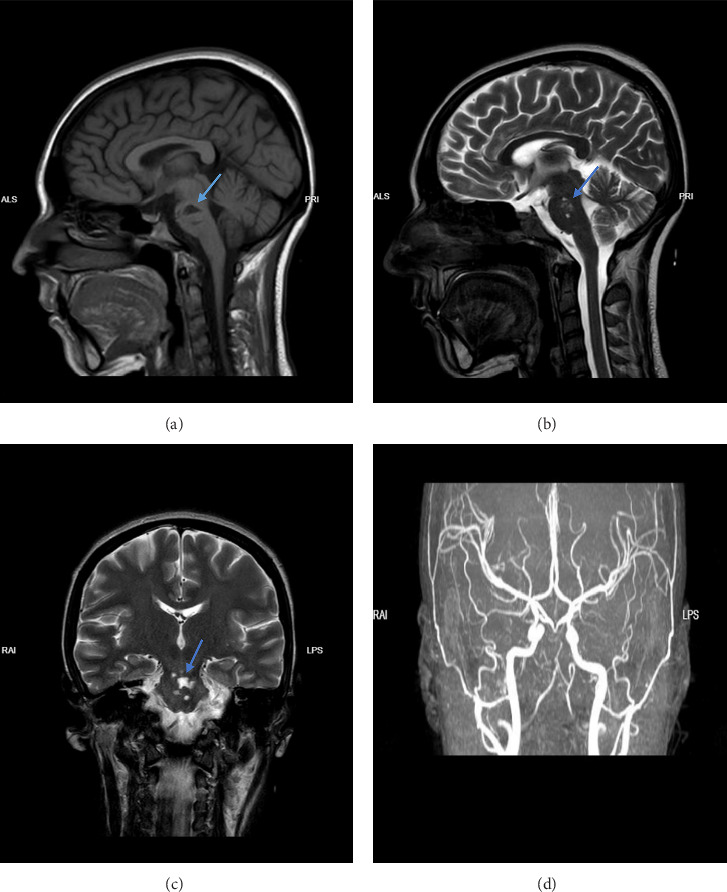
T1-weighted MRI of brain shows hyposignal areas in pons suggestive of lacunar infarction in sagittal plane. (a) T2-weighted MRI shows hypersignal areas in pons suggestive of infarction in sagittal (b) and coronal (c) planes. Brain MRA shows fetal origin of posterior cerebral arteries (PCA) bilaterally. Vertebral artery hypoplasia (VAH) was also noted (d).
